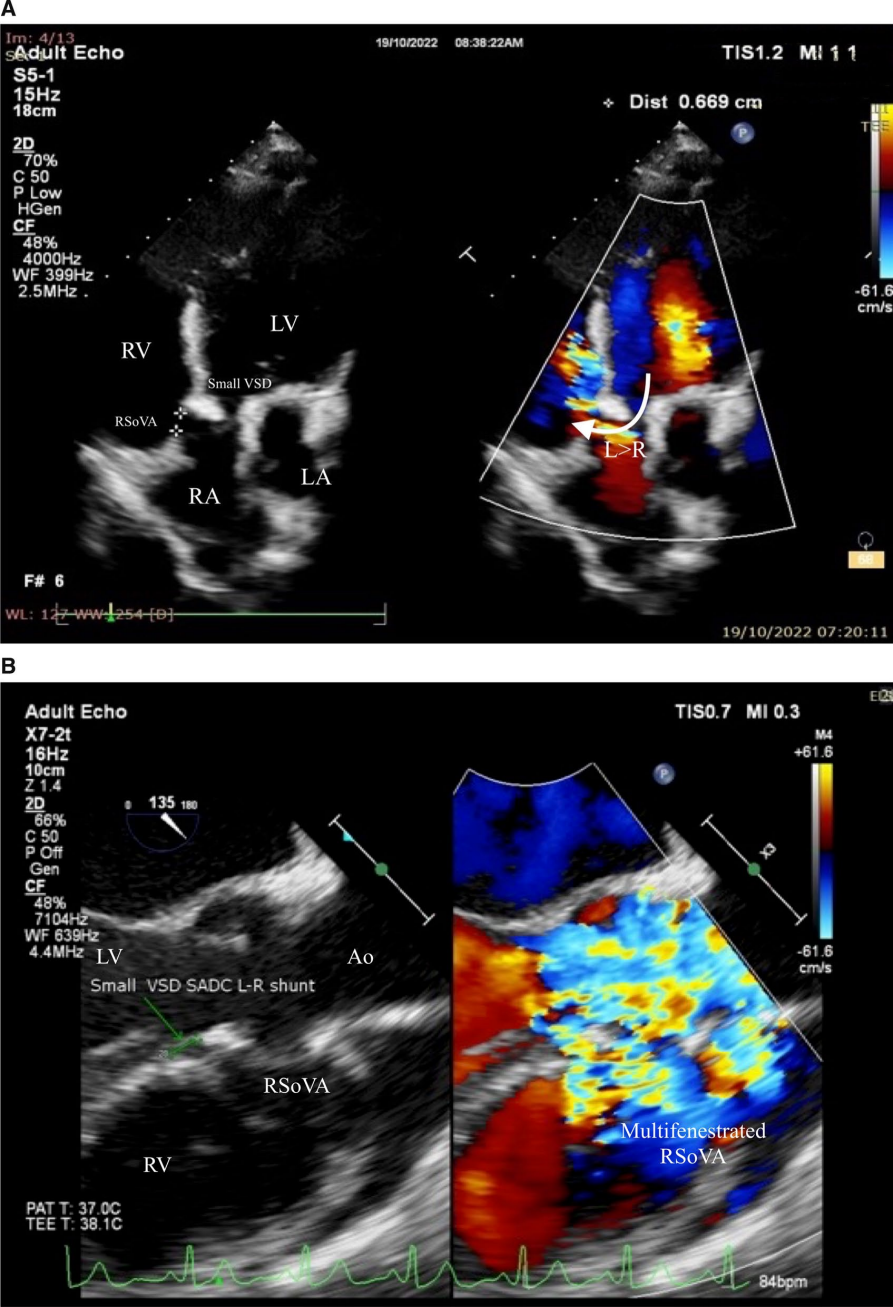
Transthoracic echocardiography presented A a small VSD sub aortic ventricular septal defect with a left-to-right shunting (arrow), viewed from an apical 5 chamber view; B ME PLAX view on transoesophageal echocardiography. Ao = Aorta; RV = Right ventricle; LV = Left ventricle; LA = Left atrium; L = Left; R = Right; RSoVA: Ruptured sinus of Valsalva aneurysm; VSD = Ventricular septal defect; SADC = Sub aterial doubly committed
